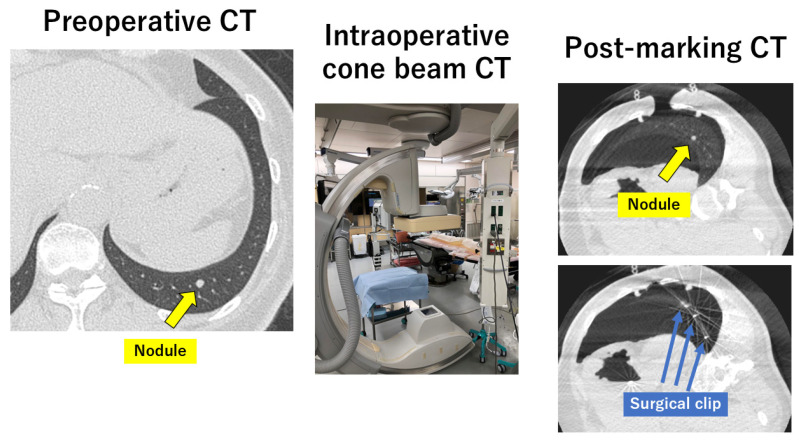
Intraoperative marking with cone beam computed tomography (CBCT). NIR: near-infrared.
